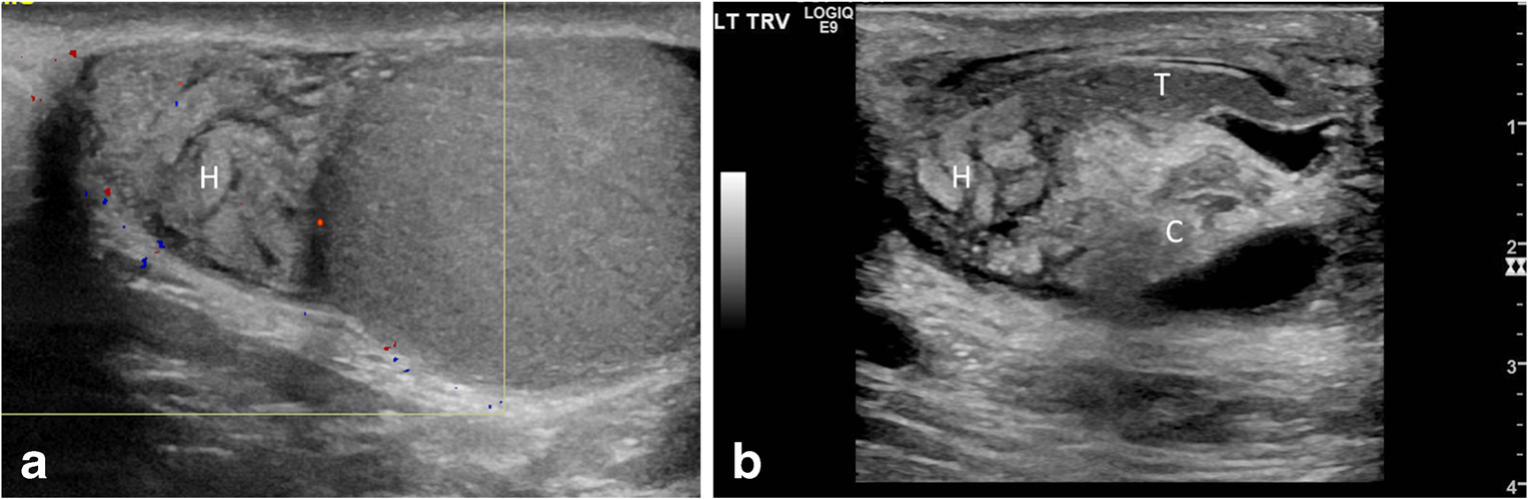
Epididymal enlargement without hyperemia. a Color Doppler longitudinal US image of the epididymal head and testis in a 12-year-old boy who woke with acute left testicular pain and nausea. Image shows an enlarged epididymal head (*H*) with a swollen lobular appearance and lack of intrinsic color flow. The intratesticular flow was decreased. He underwent emergent bilateral orchiopexy for a complete left torsion, and a bell clapper deformity was appreciated. Even though the epididymis was enlarged, it was avascular, thus ruling out epididymitis. b Gray-scale longitudinal US image of the epididymal-cord complex in a 7-year-old boy with 3 days of intermittent left testicular pain shows an edematous epididymal head (*H*) with a tangled echogenic cord (*C*) while the epididymal tail (*T*) appears relatively uninvolved. His left testis, however, showed somewhat decreased flow and he was diagnosed with partial torsion. A 360° twist was found during orchiopexy along with bilateral classic bell clapper deformity
